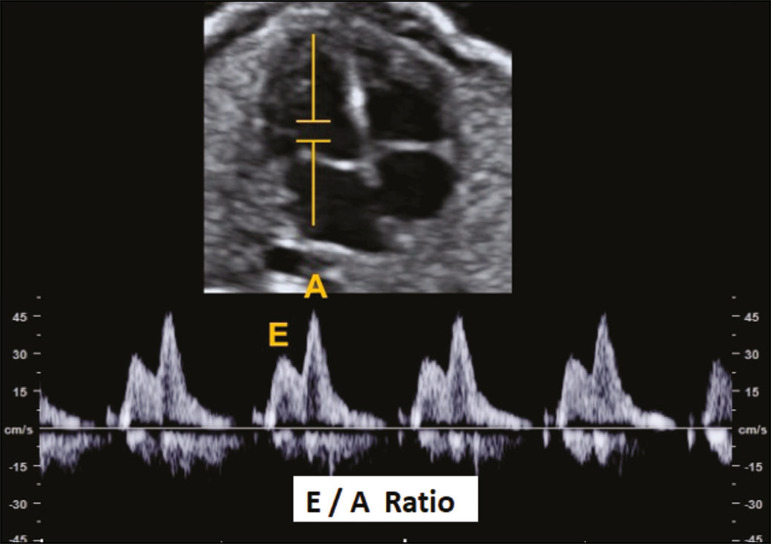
Spectral Doppler imaging showing the E and A waves through the fetal heart mitral valve.
